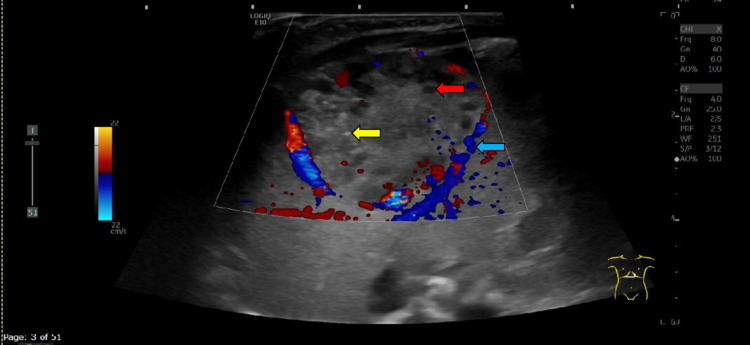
Abdominal ultrasonography for evaluation of the lesion in the left lobe liver. A transverse sonogram demonstrates a well-circumscribed oval-shaped heterogeneously hypoechoic mass with cystic spaces (red arrow), small hyperechoic structures (yellow arrow) representing tiny spots of calcification, and increased peripheral vascularity in the left lobe liver (blue arrow).
